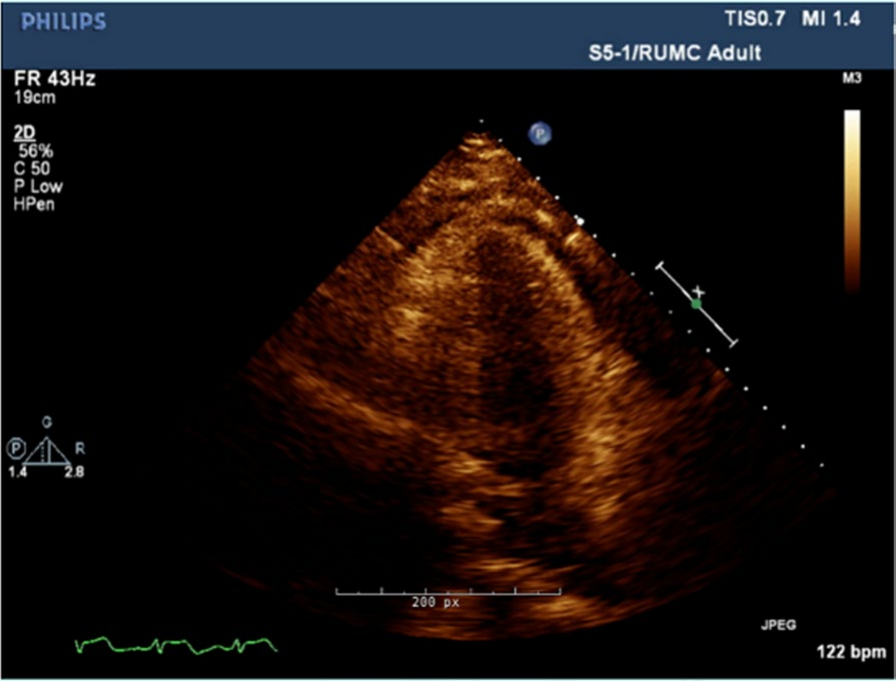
Transthoracic echocardiogram with mild pericardial effusion
